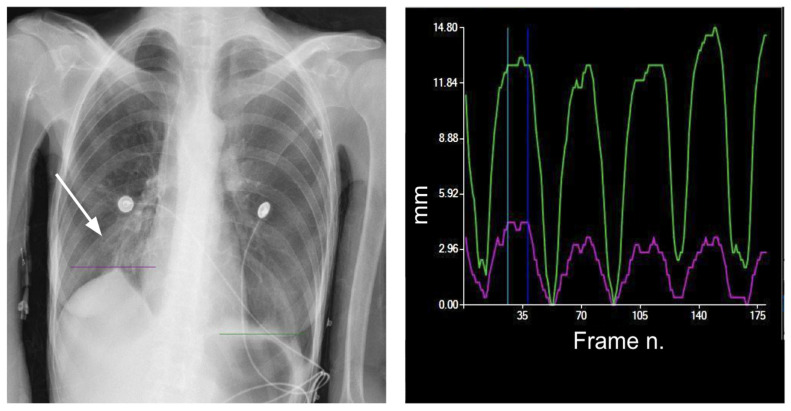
Impaired motility of the right diaphragm due to fibrotic alterations. Morphological evaluation of the X-ray exam shows an elevated and stapled right diaphragm with fibrotic bands in the lower right field (arrow). Purple line = right diaphragm; green line = left diaphragm.
